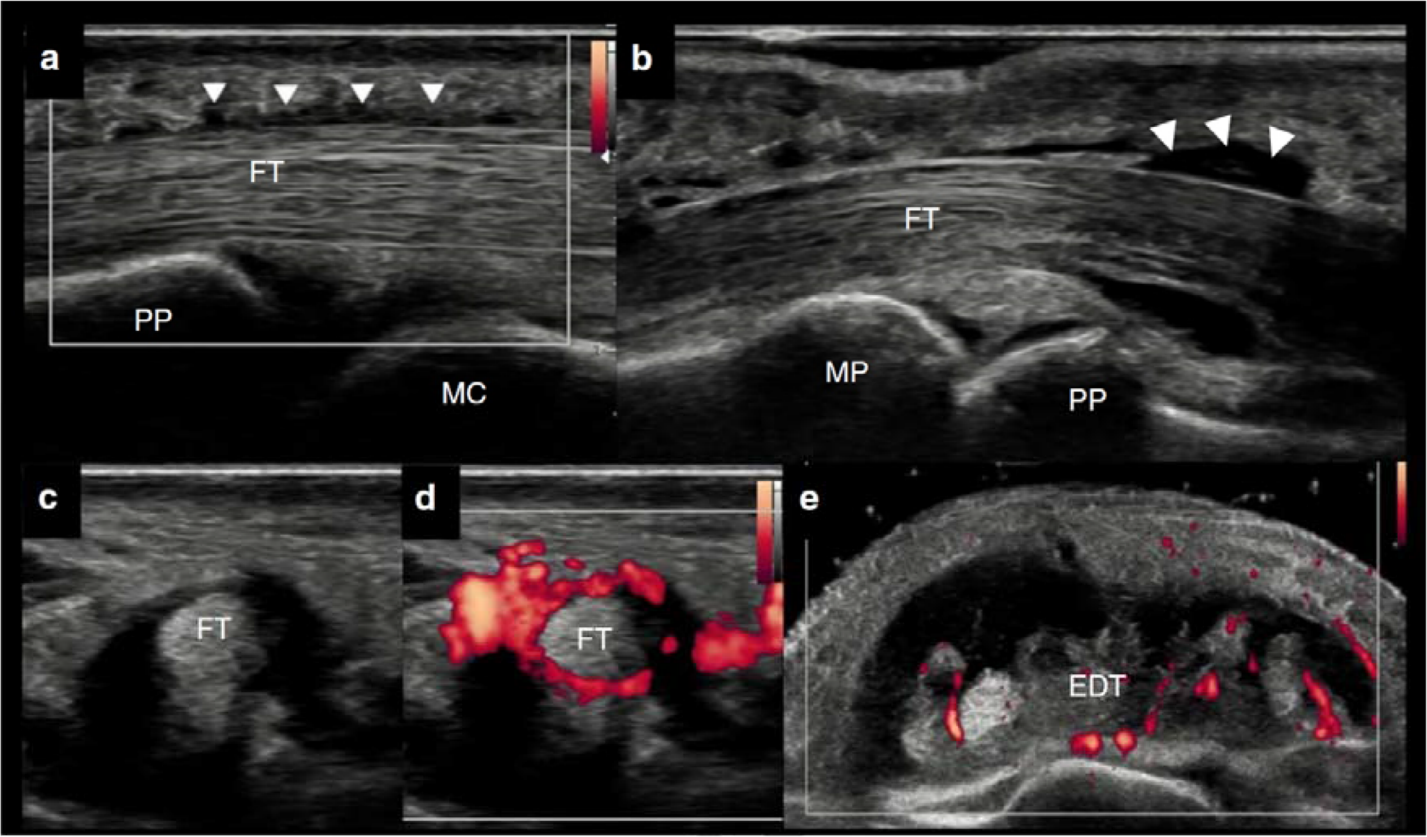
a–d Volar scan of the digit of the hands by ultrasonography. a–b Longitudinal view. Mild tenosynovitis (white arrowhead) of the flexor tendon (FT) in a psoriasis patient with arthralgia. c–d Transverse view. Active tenosynovitis of the flexor tendon in an early psoriatic arthritis. Dorsal scan of the wrist. e Active tenosynovitis of the extensor digitorum tendon (EDT) in an established psoriatic arthritis
